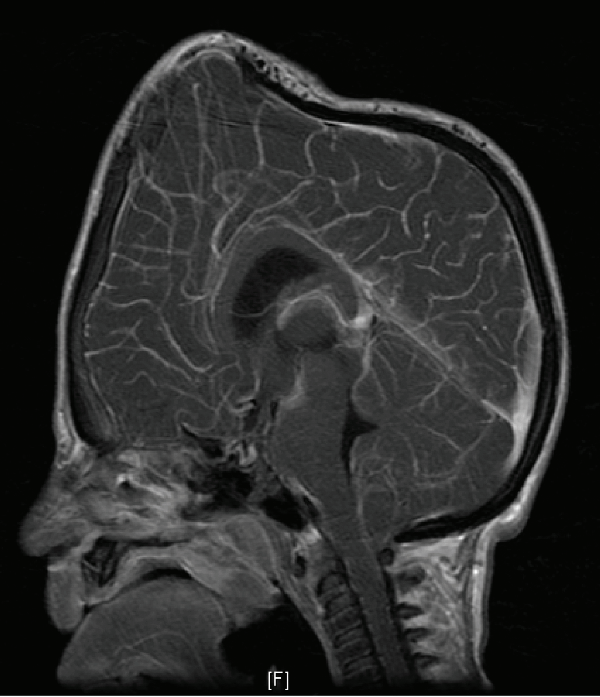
MRI (TI sagittal with contrast) showing Chiari type 1 malformation, upward bulging of frontal lobes towards the bregma, shallow anterior cranial fossa and extensive subcutaneous venous channels.
